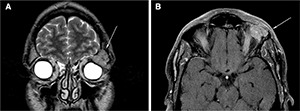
Magnetic resonance imaging: Coronal T2 TSE sequence (A) and coronal SE T1 sequence with fat suppression (B), showing expansive lesion of probable bone origin with intraorbital development, which appears isointense in T2 and shows contrast enhancement on T1 fat-suppressed sequence (white arrows).
